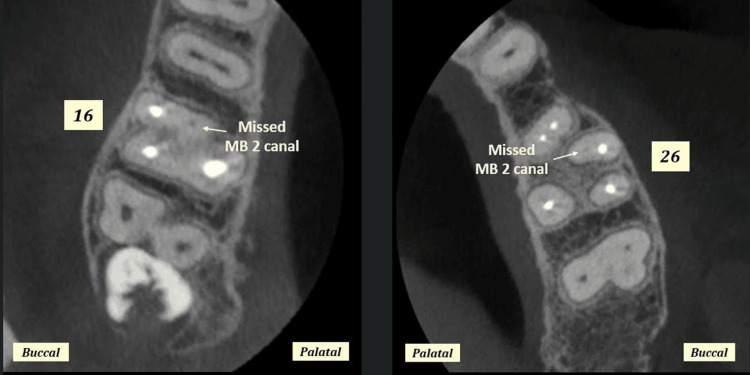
CBCT images showing missed mesiobuccal root canal configurations in axial sections of right and left maxillary first molars at cervical root third level. MB: mesiobuccal; MB2: second mesiobuccal canal; CBCT: cone beam computed tomography
